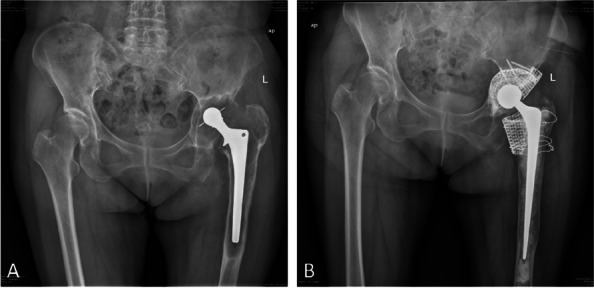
Preoperative (A) and postoperative (B) pelvic X-ray of one selected case
